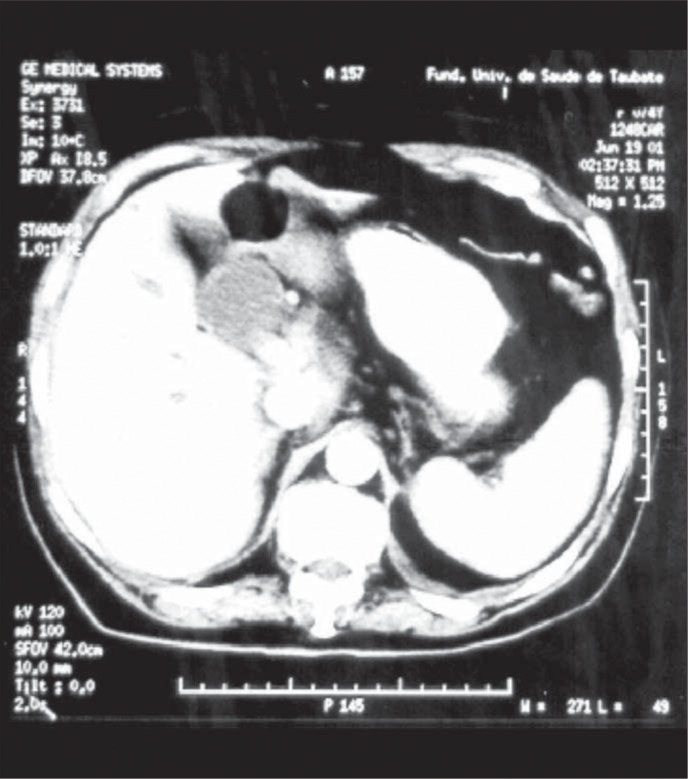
Computed tomography scan of the patient, showing common bile duct that is very much dilated.
